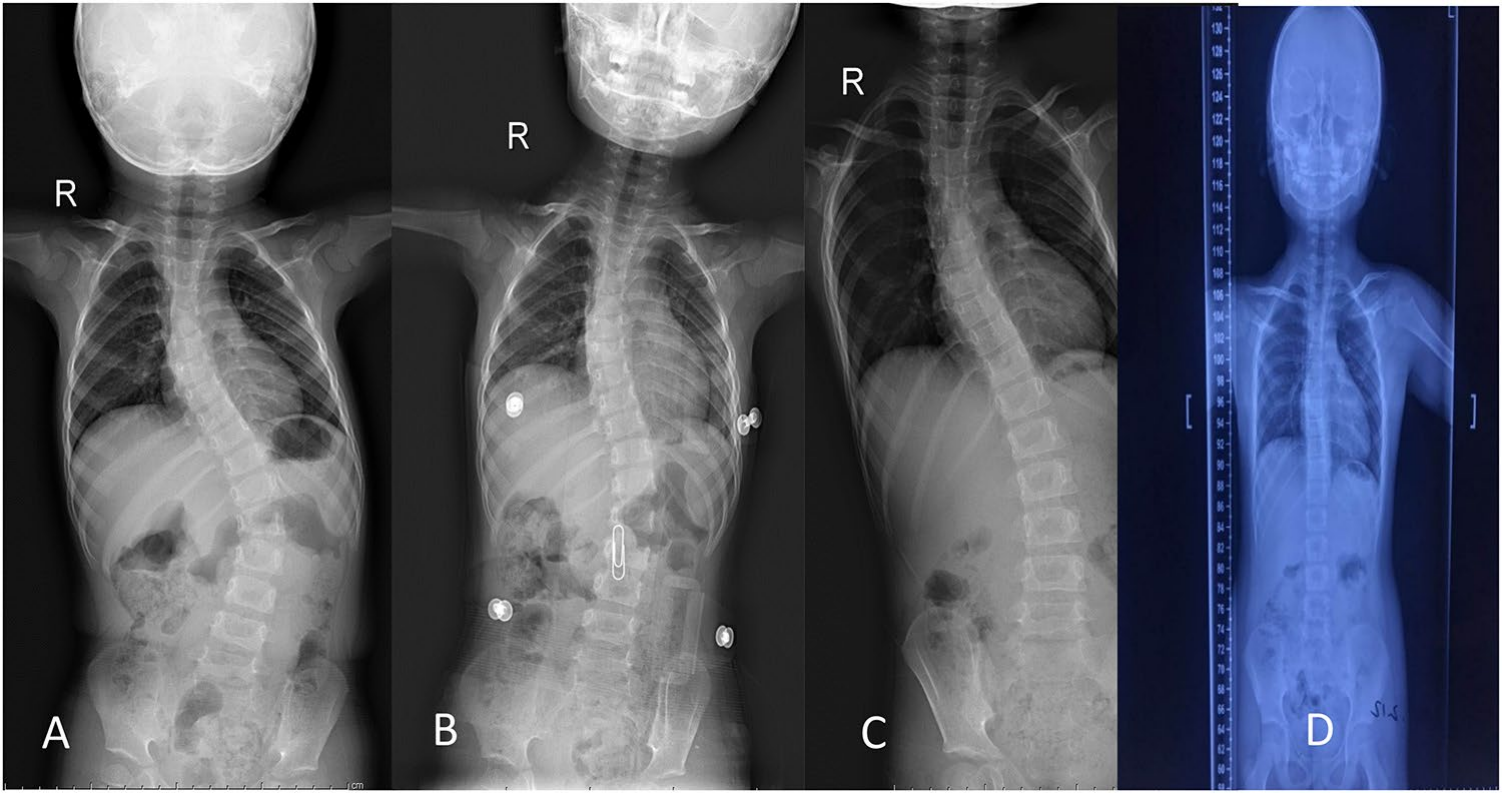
A 31-month-old boy diagnosed with idiopathic EOS with a thoracolumbar apical vertebra. X-ray obtained before brace placement (A), after initial brace placement (B), at the 2-year follow-up (C) and at the last follow-up (D).
